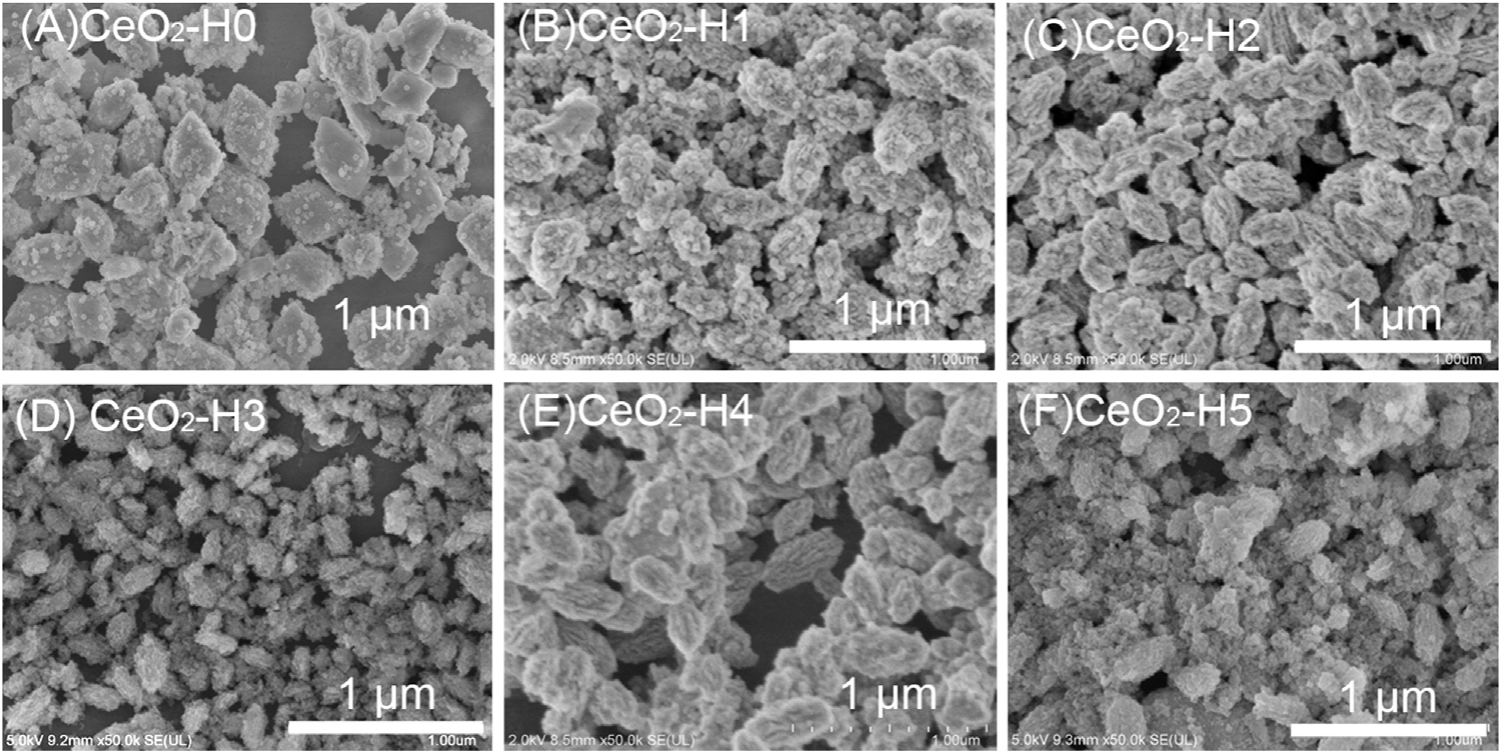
SEM morphologies of CeO_2_ prepared by the combined microwave–ultrasonic method and calcined at 500°C in air for 1 h. (A) CeO_2_-H0, (B) CeO_2_-H1, (C) CeO_2_-H2, (D) CeO_2_-H3, (E) CeO_2_-H4, (F) CeO_2_-H5.
